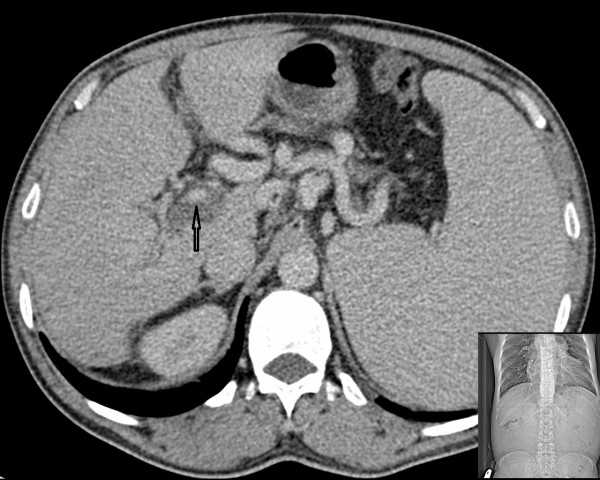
Contrast enhanced CT confirmed portal vein thrombosis formation.
